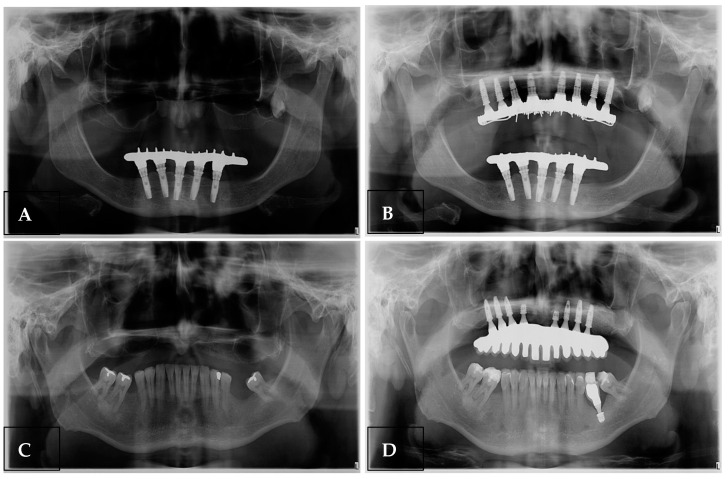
Panoramic radiographs of two clinical cases, (A,C) initial radiographs and (B,D) radiographs with the implants installed in the grafted areas 5 months after surgery.
